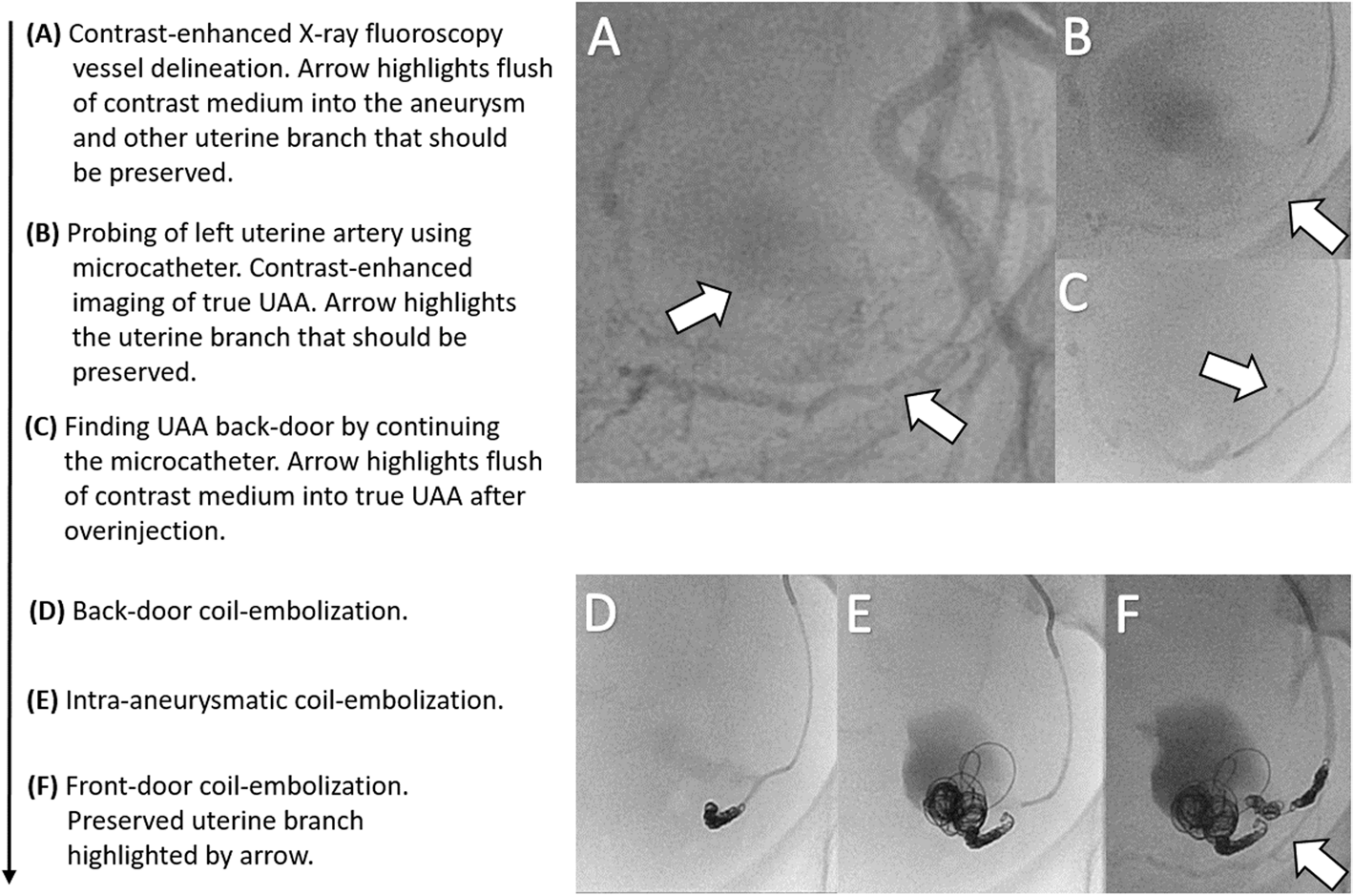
Endovascular contrast enhanced vessel delineation using X-ray fluoroscopy instead of DSA with flush of contrast medium into the aneurysm (A). After selective probing of the left uterine artery with a microcatheter better visualization of the true UAA and demarcation of another uterine branch (arrow) that should be preserved during embolization (B). Back-door of the true UAA and a little flush of contrast medium (arrow) after overinjection (C). Back-door (D), intra-aneurysm (E) and front-door (F) coil-embolization preserving the initial shown other uterine branch (F, arrow). In the final X-ray fluoroscopy, there is no contrast enhancement of the uterine vessel leading to the UAA (F). Altogether 34 coils (Cook: 2 mm Hilal, 3 mm / 8 mm / 10 mm Nester; BostonScientific: 3 mm IDC, 20 mm Interlock) were used for embolization
